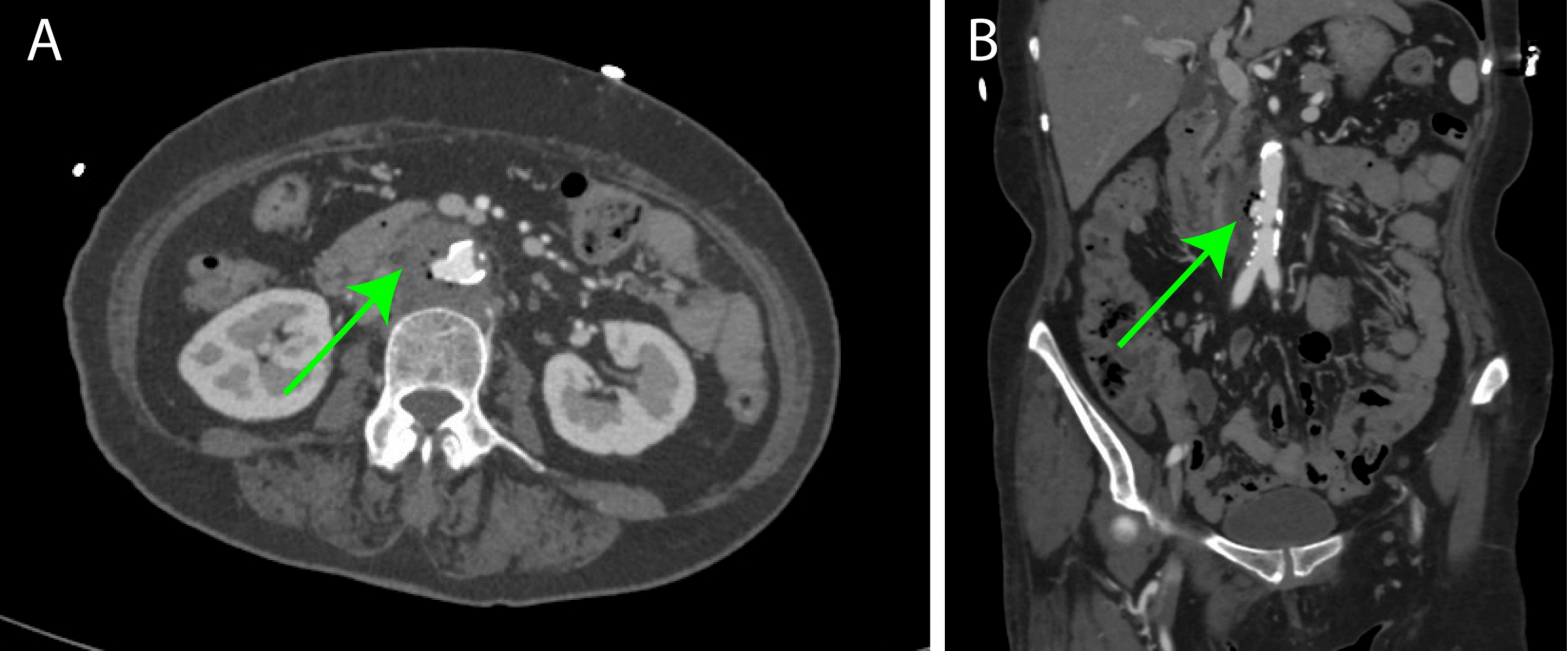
Preoperative enhanced computed tomography image (A) A contrast-enhanced CT scan revealed a penetrating atherosclerotic ulcer in a section of the abdominal aorta, with air pockets surrounding it (green arrow). (B) Coronal view.
